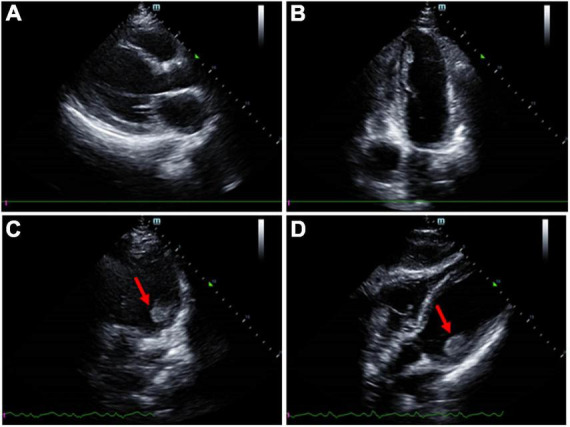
Transthoracic echocardiogram imaging of the patient. (A) Parasternal window parasternal long-axis view. (B) Apical window four-chamber view. (C,D) A mural thrombus was identified attached to the posteromedial papillary muscle.
